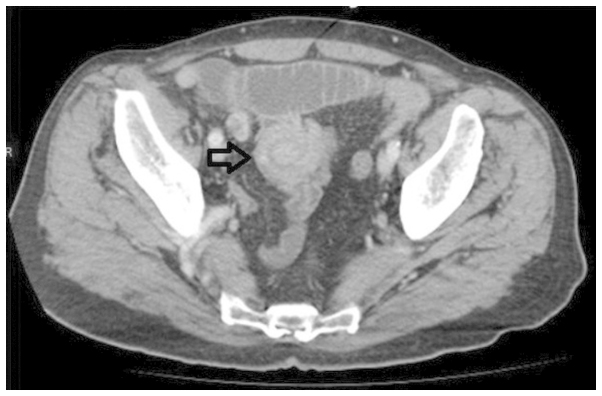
Computed tomography revealing a contrast enhanced mass (black arrow), which resembled a neuroendocrine tumor.
